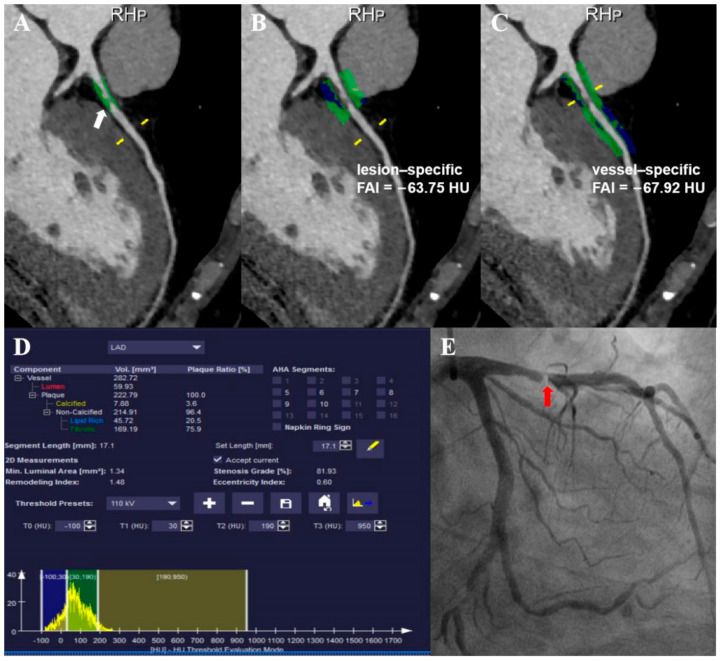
Example of a 42-year-old man with chest pain: (A) CCTA image showed a lesion analyzed with dedicated plaque analysis software proximal to the LAD with stenosis ranging between 70 and 90% (white arrow); (B,C) color-coded CPR image reveals that the mean perivascular FAIs of the lesion (lesion-specific FAI) and proximal 40 mm of LAD (vessel-specific FAI) were −63.75 HU and −67.92 HU, respectively; (D) measurement list shows the contents of various plaque components; (E) ICA showed that the stenosis degree of the lesion was about 90% (red arrow), and then FFR confirmed that the stenosis was hemodynamically significant (FFR = 0.75). CCTA, coronary computed tomography angiography; FAI, fat attenuation index; LAD, left anterior descending artery; CPR, curved planar reformation; HU, Hounsfield unit; ICA, invasive coronary angiography; FFR, fractional flow reserve.
